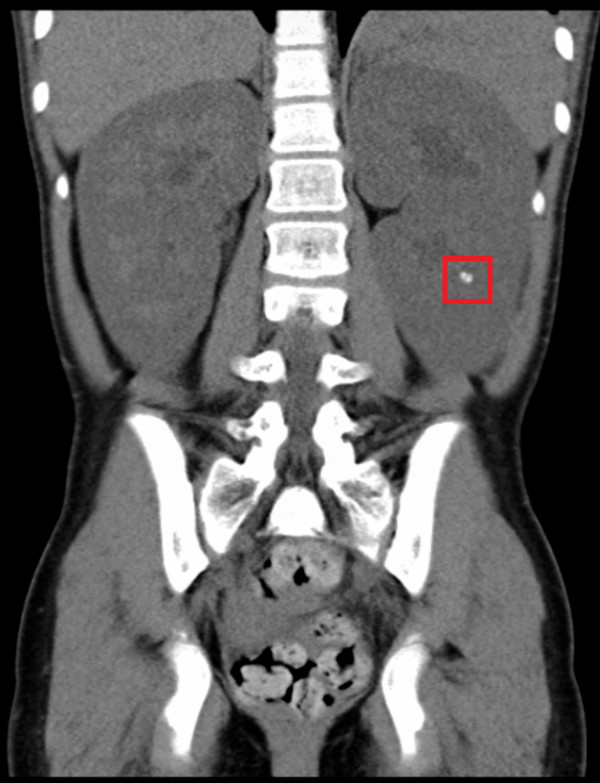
Renal manifestations on CT abdomen. Bilateral nephromegaly (Greater than 2 standard deviation for age), and nephrocalcinosis (in red box).
